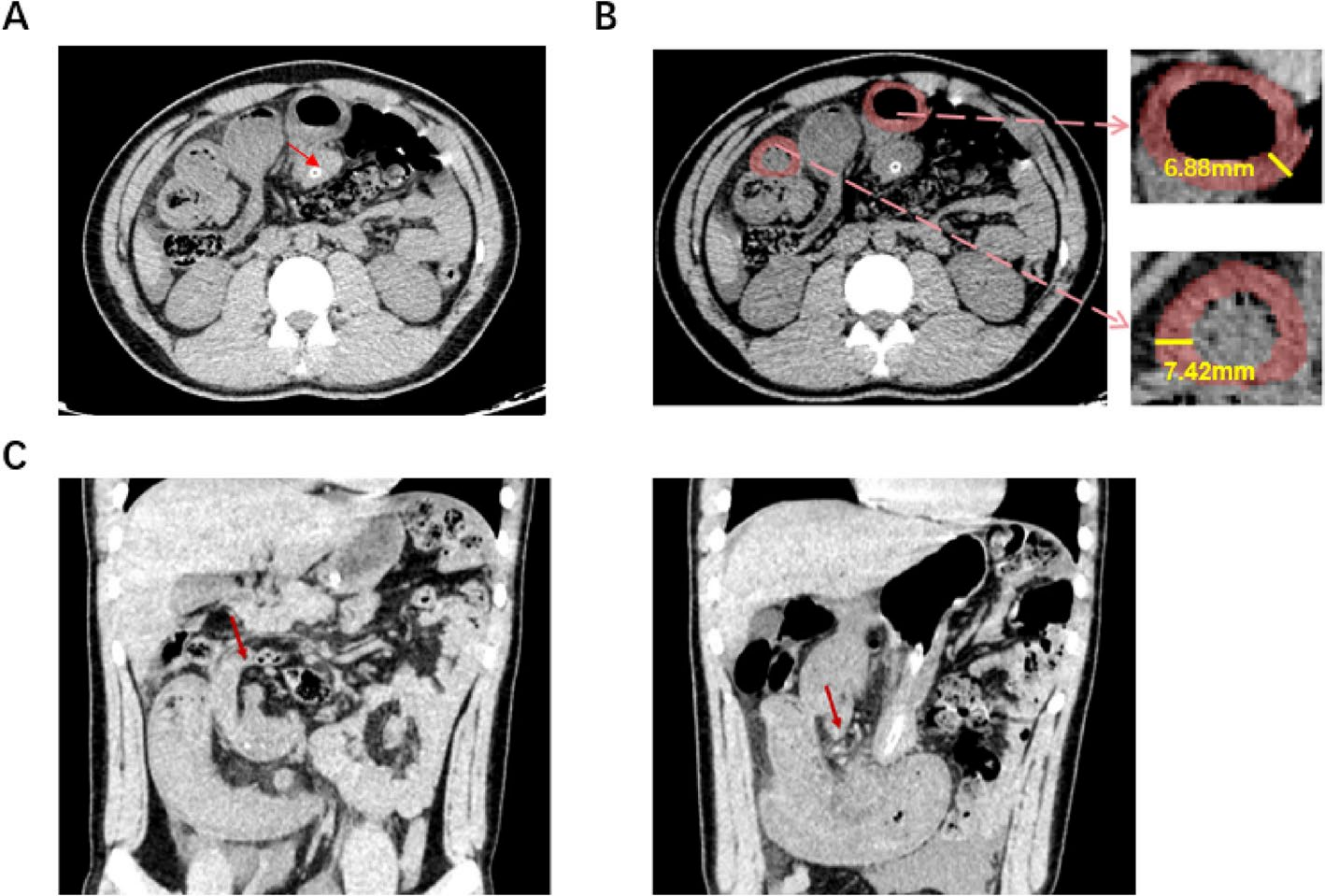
Preoperative abdominal CT findings of an internal hernia through a congenital mesenteric defect posterior to the gastric antrum with strangulated small bowel. A Axial CT showing an abnormally positioned small bowel loop with focal dilatation (red arrow). B Axial CT demonstrating the herniated small bowel loop with thickened bowel wall and luminal narrowing. Magnified views reveal bowel wall edema, with measured thicknesses of 6.88 mm and 7.42 mm. C Coronal CT further showing the terminal ileum herniated into the right upper abdominal cavity (red arrow), accompanied by obstruction and edema
